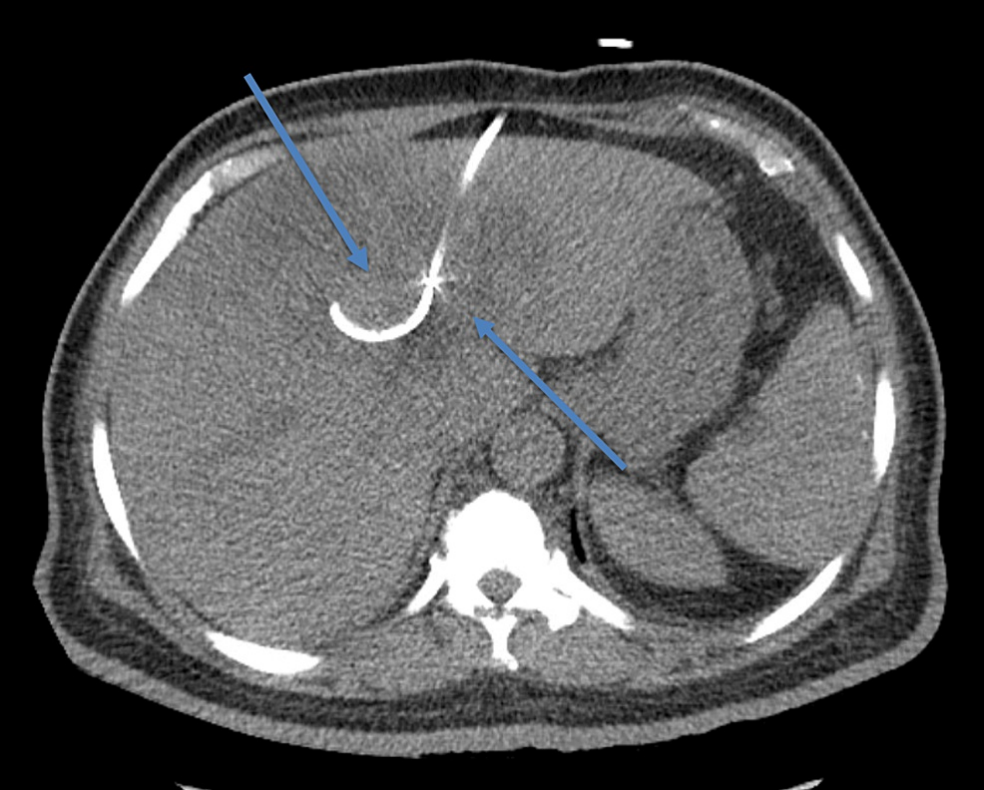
CT Abdomen with and without intravenous (IV) contrast. A large, multiloculated hypodense lesion in the liver is compatible with abscesses treated with a pigtail drainage catheter.
